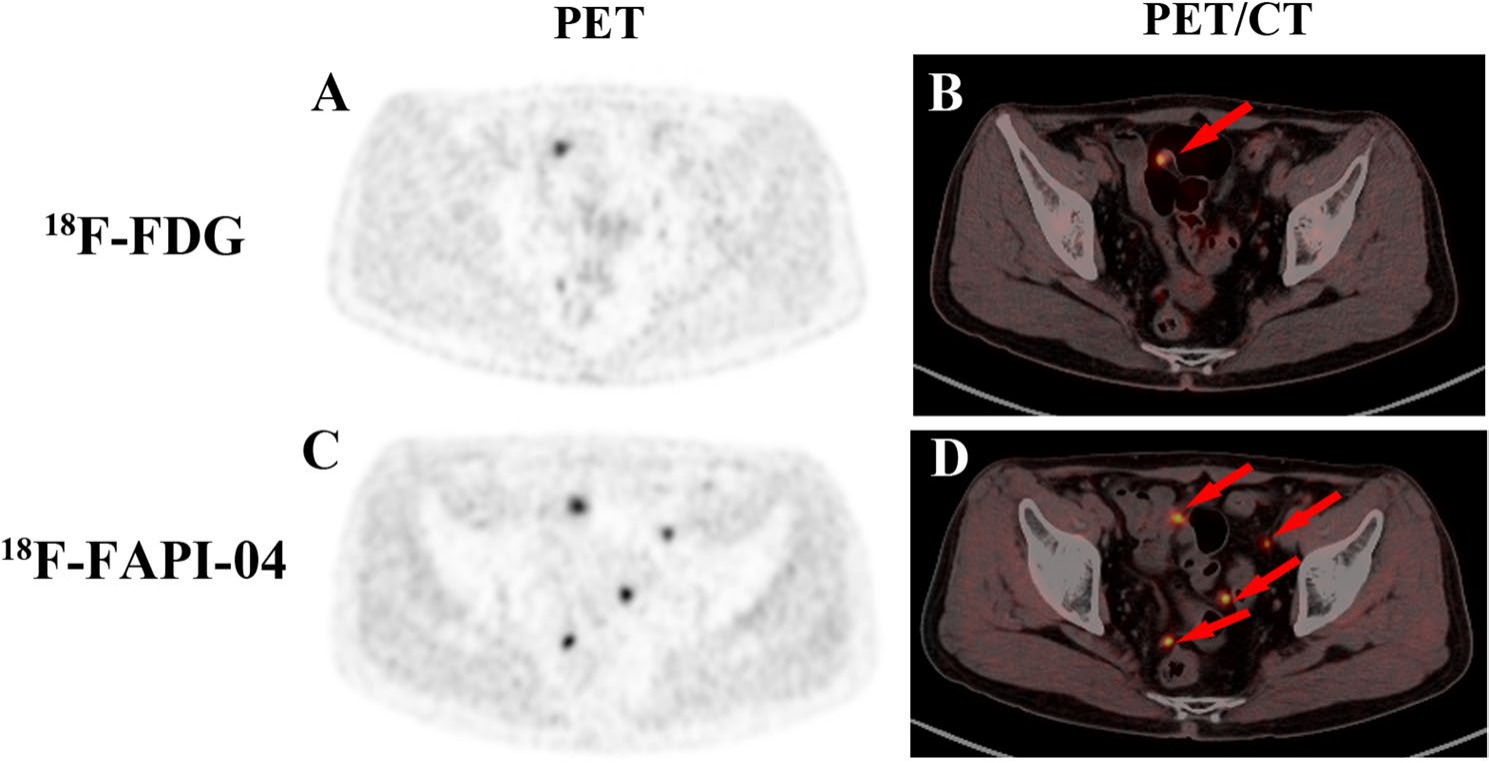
A 62-year-old male patient with pancreatic cancer-associated PM underwent PET/CT imaging for evaluation. ^18^F-FAPI-04 PET exhibited markedly higher uptake in pelvic lesions than ^18^F-FDG PET and identified additional metastatic foci
